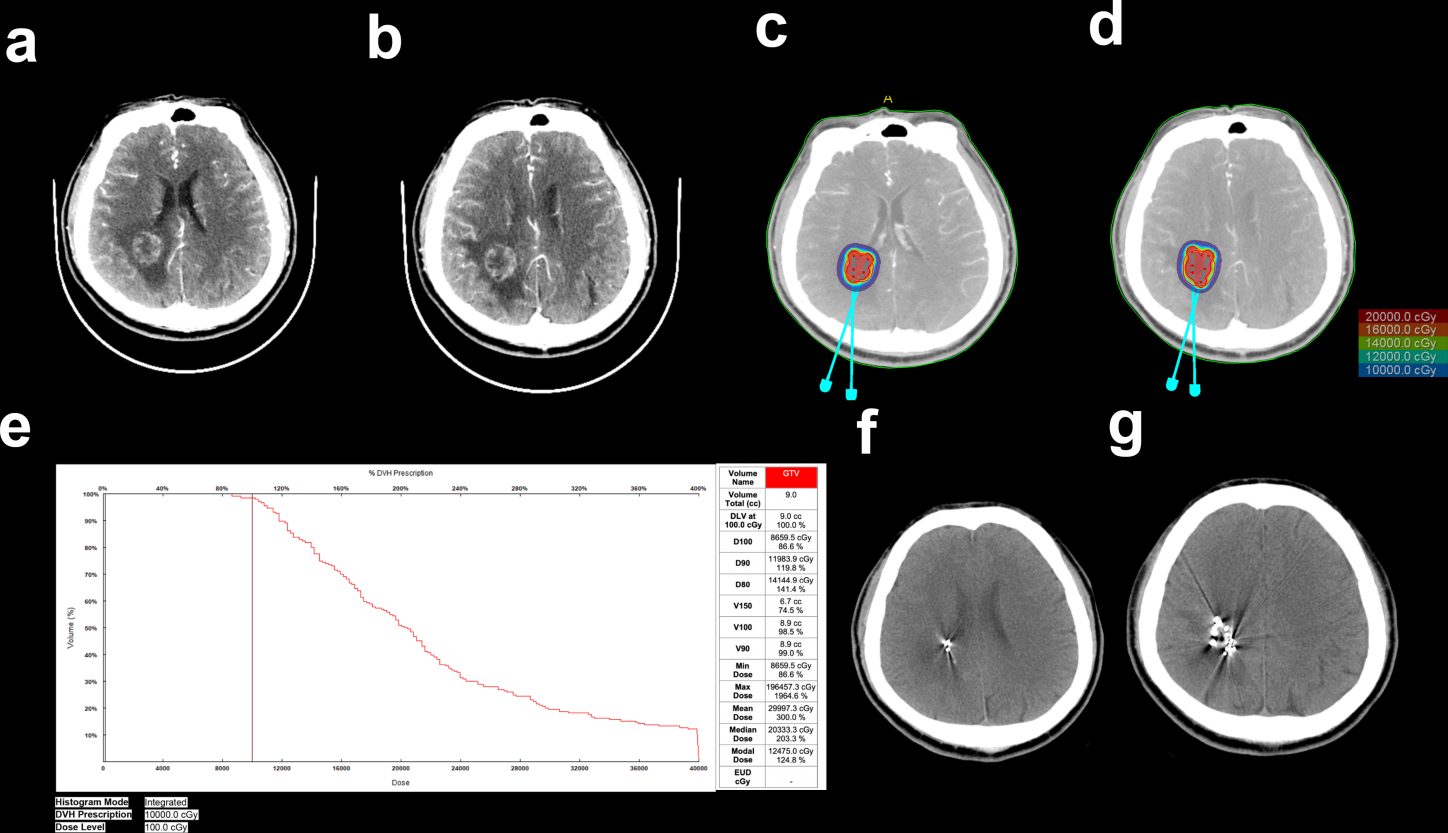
Implantation of ^125^I seeds with the guidance of TPS. (A, B) Enhanced CT images of BMs resulting from NSCLC. (C, D) Needle paths were designed with TPS. (E) Preimplantation dose volume histogram (DVH) of GTV. (F, G) CT images of the brain after ^125^I seed implantation.
